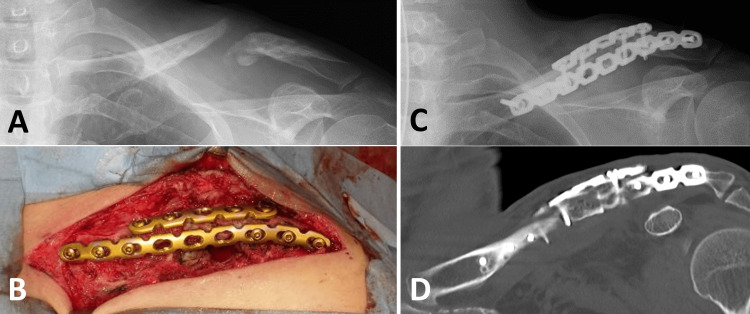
Reconstruction surgery for nonunion X-ray imaging showed protrusion of the proximal fragment toward the skin (A). Both the photograph and X-ray demonstrated double plate fixation and bone grafting using a tricortical block and cancellous chips (B, C). Computed tomography indicated adequate compression between the tricortical block and the fracture fragments (D).
